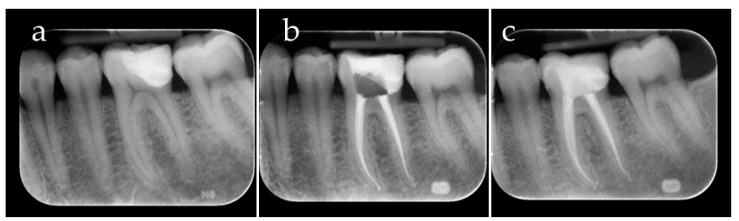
(a) A pre-operative radiograph of the first mandibular molar; (b) a radiograph after the endodontic treatment; (c) a post-operative radiograph after the indirect restoration cementation.
